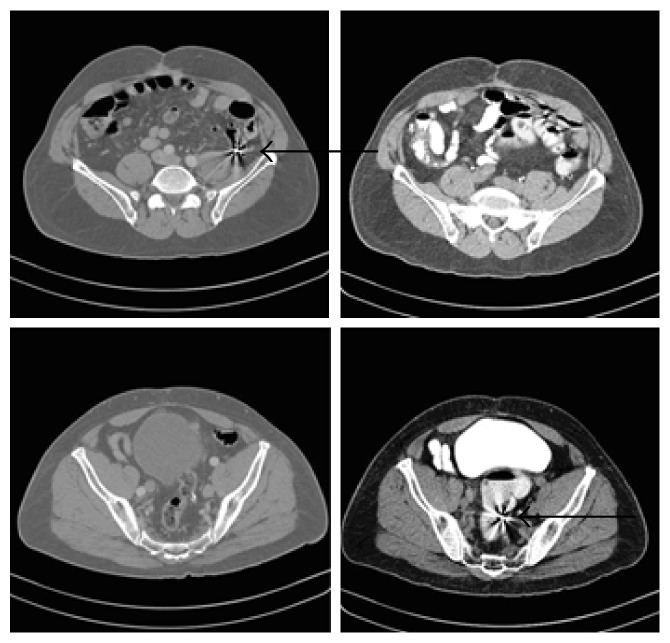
Abdominal computed tomography (CT) shows bullet fragments in the endoluminal descending colon (top arrow). Bullet migration located in the descending colon upon initial CT scan is now located in the rectal ampulla (bottom arrow).
